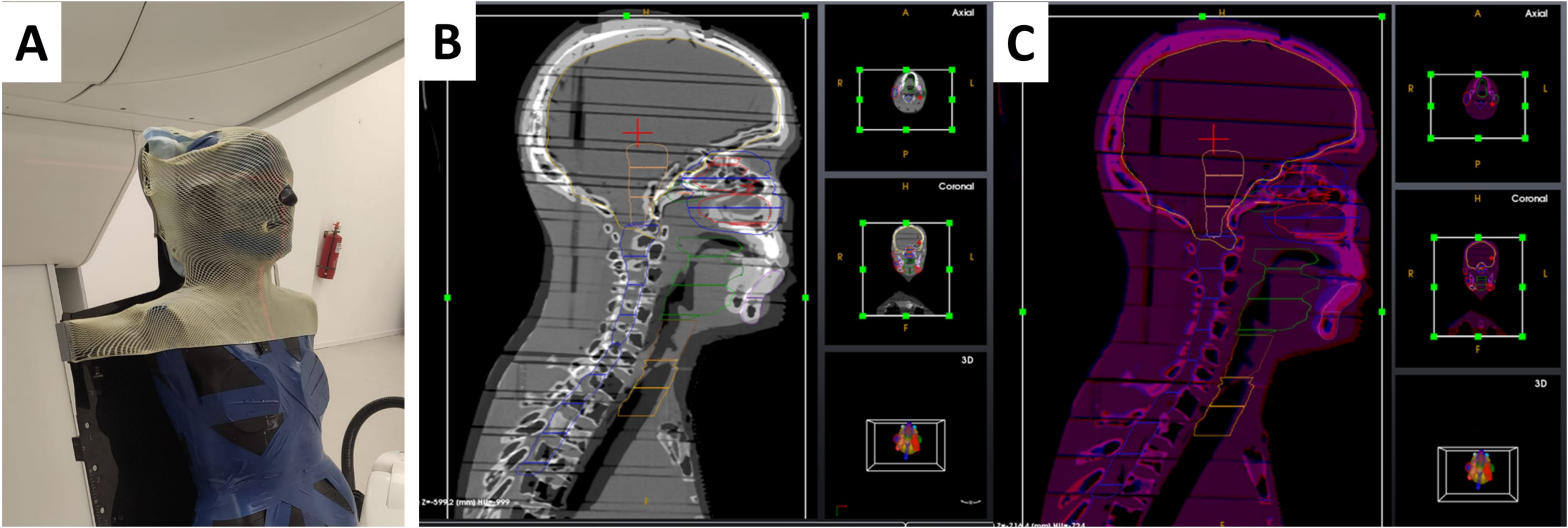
Patient positioning and imaging system calibration and setup for end-to-end testing. (A) CT image acquisition setup for head and neck case. (B, C) 3D/3D registration of the head and neck area before (B) and after (C) alignment.
